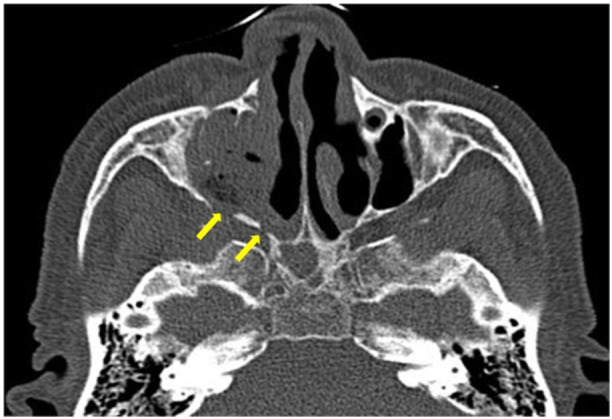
Axial noncontrast computed tomography (CT) image in bone window demonstrating involvement of the bony margins of the right pterygopalatine fossa. Arrows indicate areas of cortical erosion.
